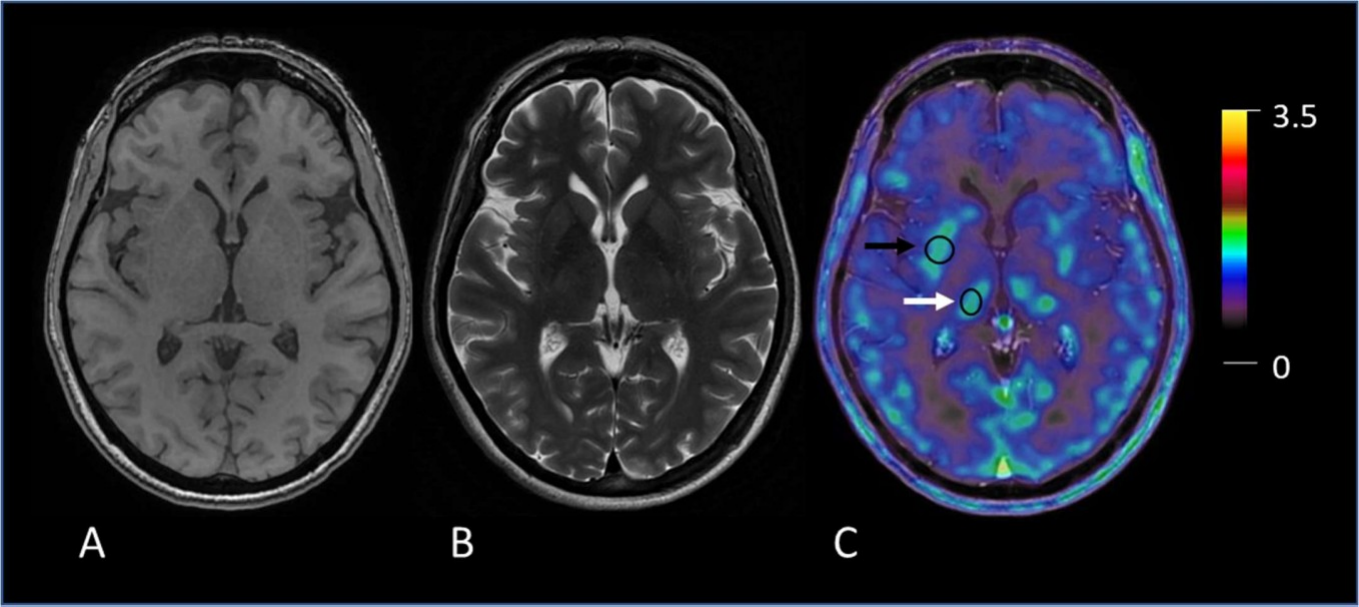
Example of measurement of 18F-FET uptake in anatomic brain structures. A T1-weighted (T1w) MR image, B T2-weighted (T2w) MR image, C fused image PET/MR image. Examples of two volumes-of-interest (VOI) for 18F-FET uptake calculation in physiological structures: putamen (black arrow) and thalamus (white arrow).
